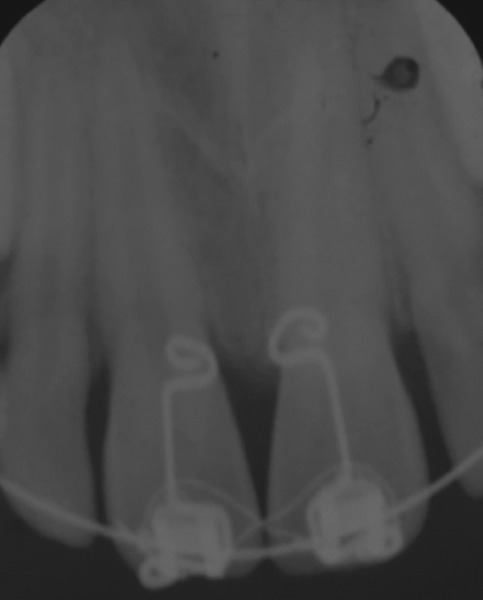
Posttreatment IOPA radiograph showing almost parallel roots of central incisors
